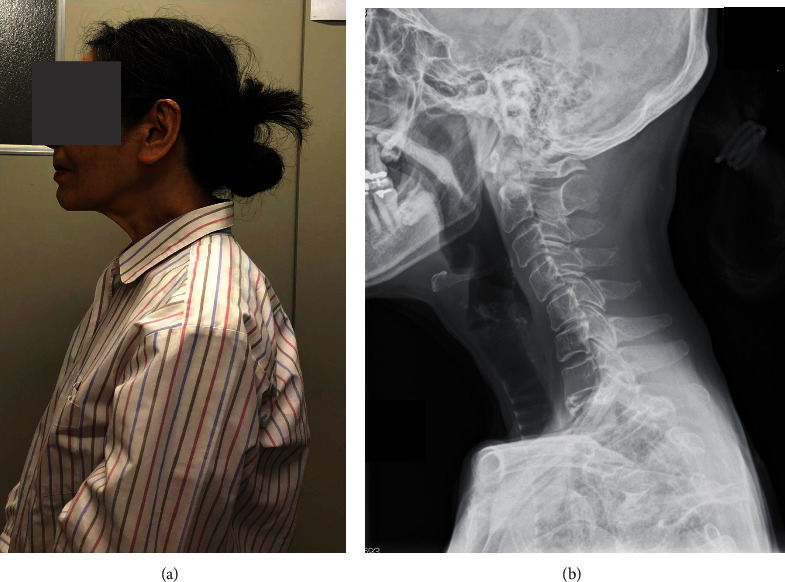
Lateral view of the patient's neck (a) and her cervical lateral radiograph (b) seven months after the 1st AR-PT. (a) The patient's neck posture was normal, and the muscle bulging had disappeared. (b) Forward inclination of her cervical spine was improved.
